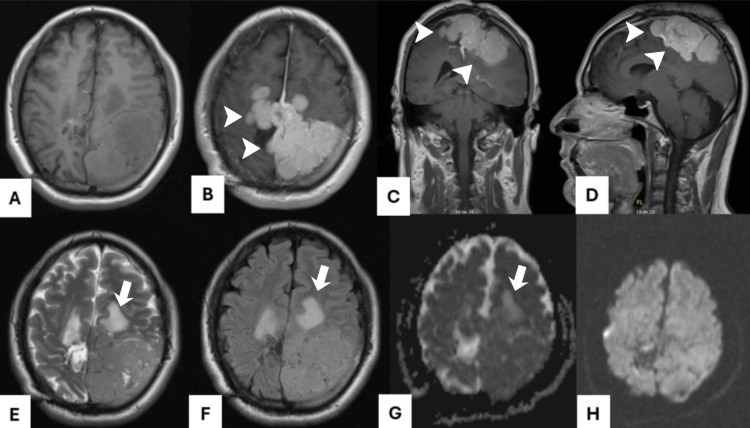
Predominant lesion in the middle third of falx. Tumor appears isointense on (A) T1, (E) T2, and (F) FLAIR, with a multilobulated morphology and well-defined borders, presenting small central heterogeneous areas. (B, C, D) Considerable enhancement was observed after contrast administration. (G) On ADC, it appears predominantly isointense (H) as in DWI. Arrowheads: Enhancing lesion. Arrows: Peritumoral edema. FLAIR: fluid-attenuated inversion recovery; ADC: apparent diffusion coefficient; DWI: diffusion-weighted imaging
